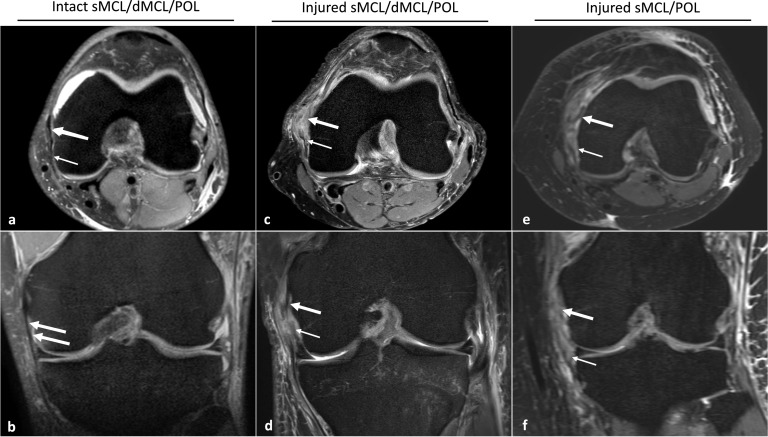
T2 weighted axial and coronal MRI sequences. Images (a and b) show an intact MCL complex. In the coronal b sequence the white arrows point to the intact POL and in the axial a sequence the large arrow points to an intact sMCL and the small arrow to an intact POL. Images (c and d) demonstrate a complete medial complex tear. In the axial c sequence the large white arrow points to the sMCL lesion and the small white arrow to the POL lesion. In the coronal sequence the large white arrow points to the sMCL lesion and the small white arrow to the dMCL lesion. In Images (e and f) one may observe coronal and axial sequences of a proximal rupture of the sMCL (large white arrows) and a POL lesion (small white arrows)
